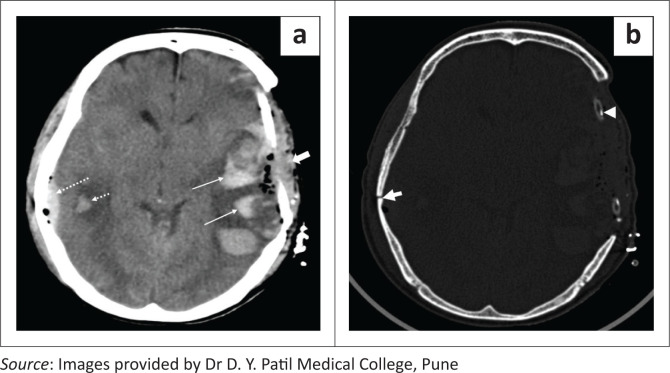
Axial soft tissue window CT brain image (a) in a patient with left decompressive fronto-parietal craniotomy reveals subgaleal hemorrhage (thick short white arrow) with postoperative pneumocephalus and multiple hyperdense intraparenchymal hemorrhages in the left fronto-parietal region with surrounding oedema (long white arrows). Post-operative drain tube is noted at the craniectomy site on image b, bone window CT image (arrowhead). Also note the small lentiform epidural haemorrhage and intraparenchymal hematoma on the right side (a, white dashed arrows) associated with a fracture of the right parietal bone (b, short thick arrow).
